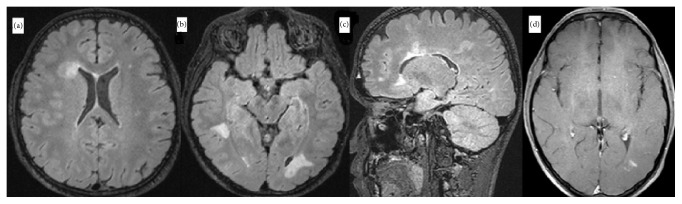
One year later. (a) Regression of tumefactive lesions is evident. (b) Axial fluid attenuation inversion recovery (FLAIR) image shows new hyperintense lesions in the bilateral parietooccipital white matter. (c) Sagittal FLAIR image demonstrates periventricular, cortical/juxtacortical demyelinating lesions. (d) T1-weighted postcontrast image shows contrast enhancement of the lesion in the left parietooccipital white matter.
